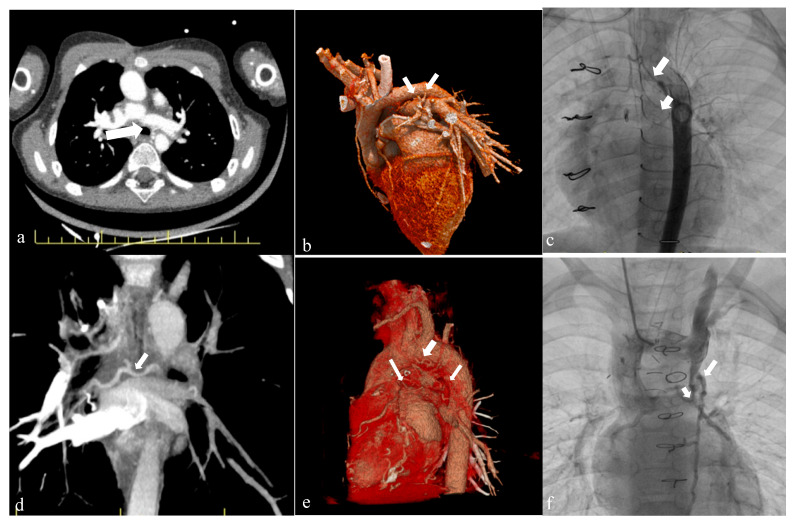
Demonstration of multiple collaterals (a–c): In a patient diagnosed with TA-VA discordance, collaterals originating from the descending aorta and arch and extending to both hila are shown with CTA and CCA. (d–f): In a patient diagnosed with VSD-PA, collaterals originating from the left innominate artery and arch are shown with CTA and CCA.
